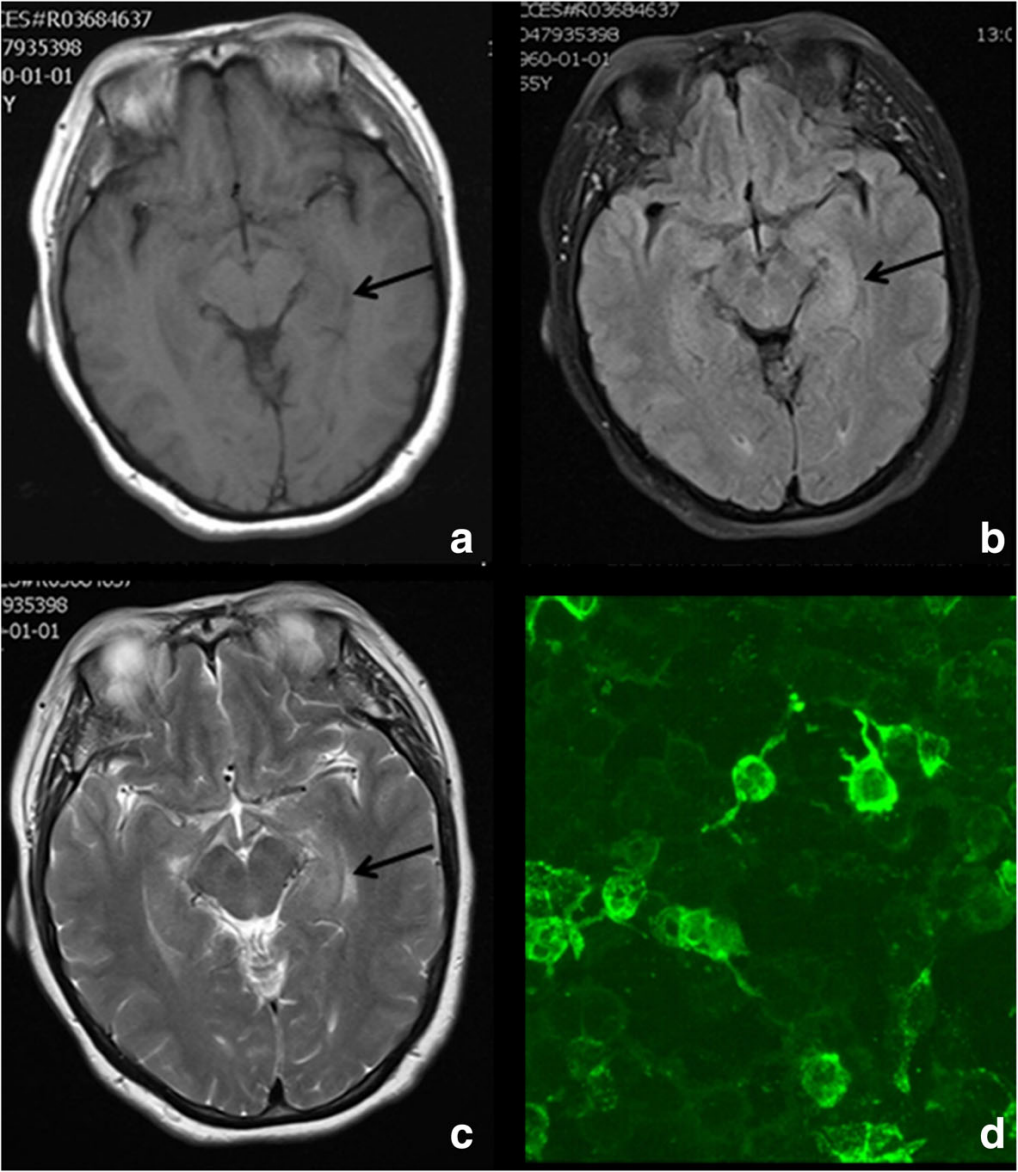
a-d. Axonal magnetic resonance imaging (MRI) scans revealed patchy lesions in the left temporal lobe and hippocampus. The lesions were represented by areas of low signal intensity on T1-weighted imaging (a) and high signal intensity on T2-weighted imaging (b) sequence and high signal intensity on the fluid-attenuated inversion recovery (FLAIR) sequence (c). Anti-AMPAR2 antibodies in the serum of the patient was positive, as tested by CBA method (d)
